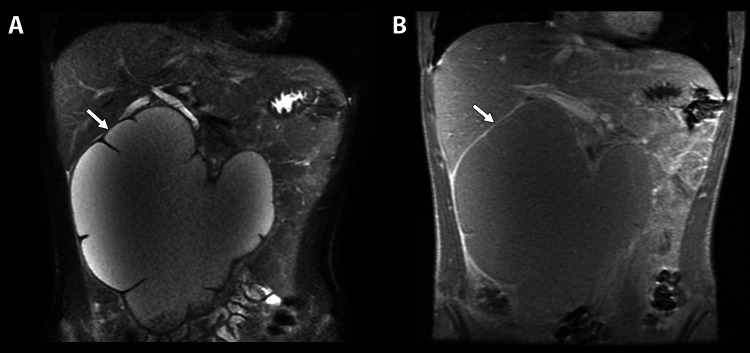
(A) Coronal T2-weighted single-shot fast spin echo (SSFSE) image demonstrating a large, lobulated, cystic mass containing internal septations along the periphery of the mass. (B) Coronal T1-weighted fast spoiled gradient-recalled echo (FSPGR) post-contrast image demonstrating the lobulated, cystic mass with visible septations and mild enhancement of the cyst wall.
